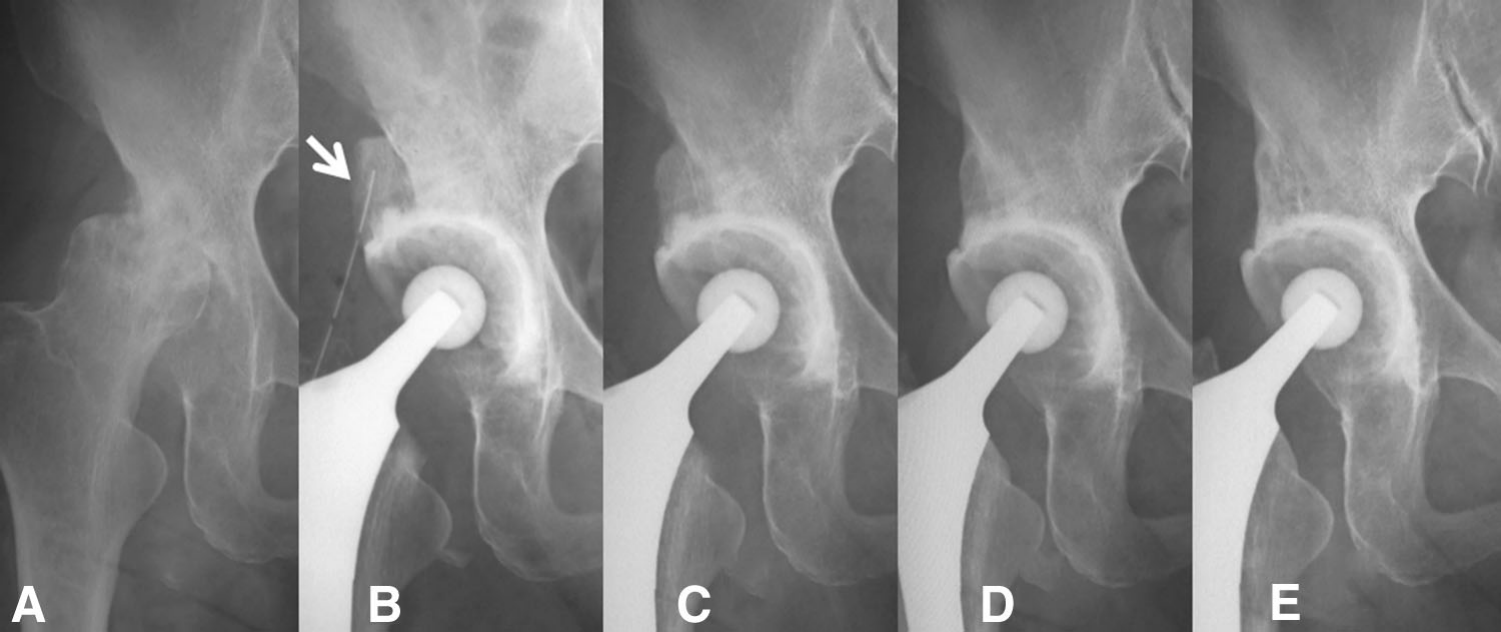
Remodeling and reorientation of the additional bulk bone grafting (arrow) with morselized bone chips for shallow acetabulum are shown. Consecutive radiographs of the left hip of a woman (age at surgery 67 years): preoperatively (A), just after THA (B), bridging trabeculation observed at 6 months (C), remodeling at 12 months (D), and reorientation at 36 months postoperatively (E).
